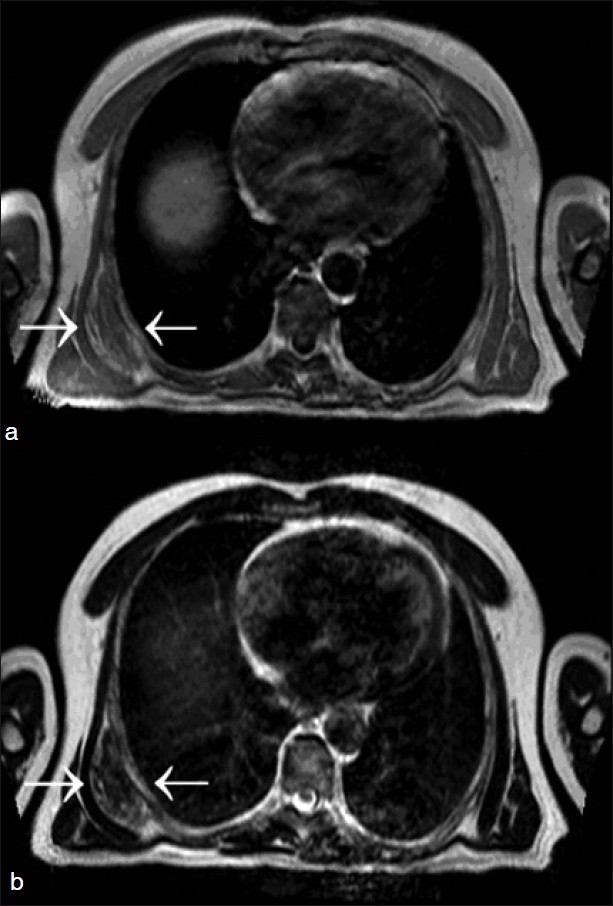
MRI of a subscapular elastofibroma from our patient is shown. (a) T1- and (b) T2-weighted images showing the typical well-defined, inhomogenous mass (arrows), with signal intensity similar to that of surrounding skeletal muscle and interlaced with areas of adipose tissue
